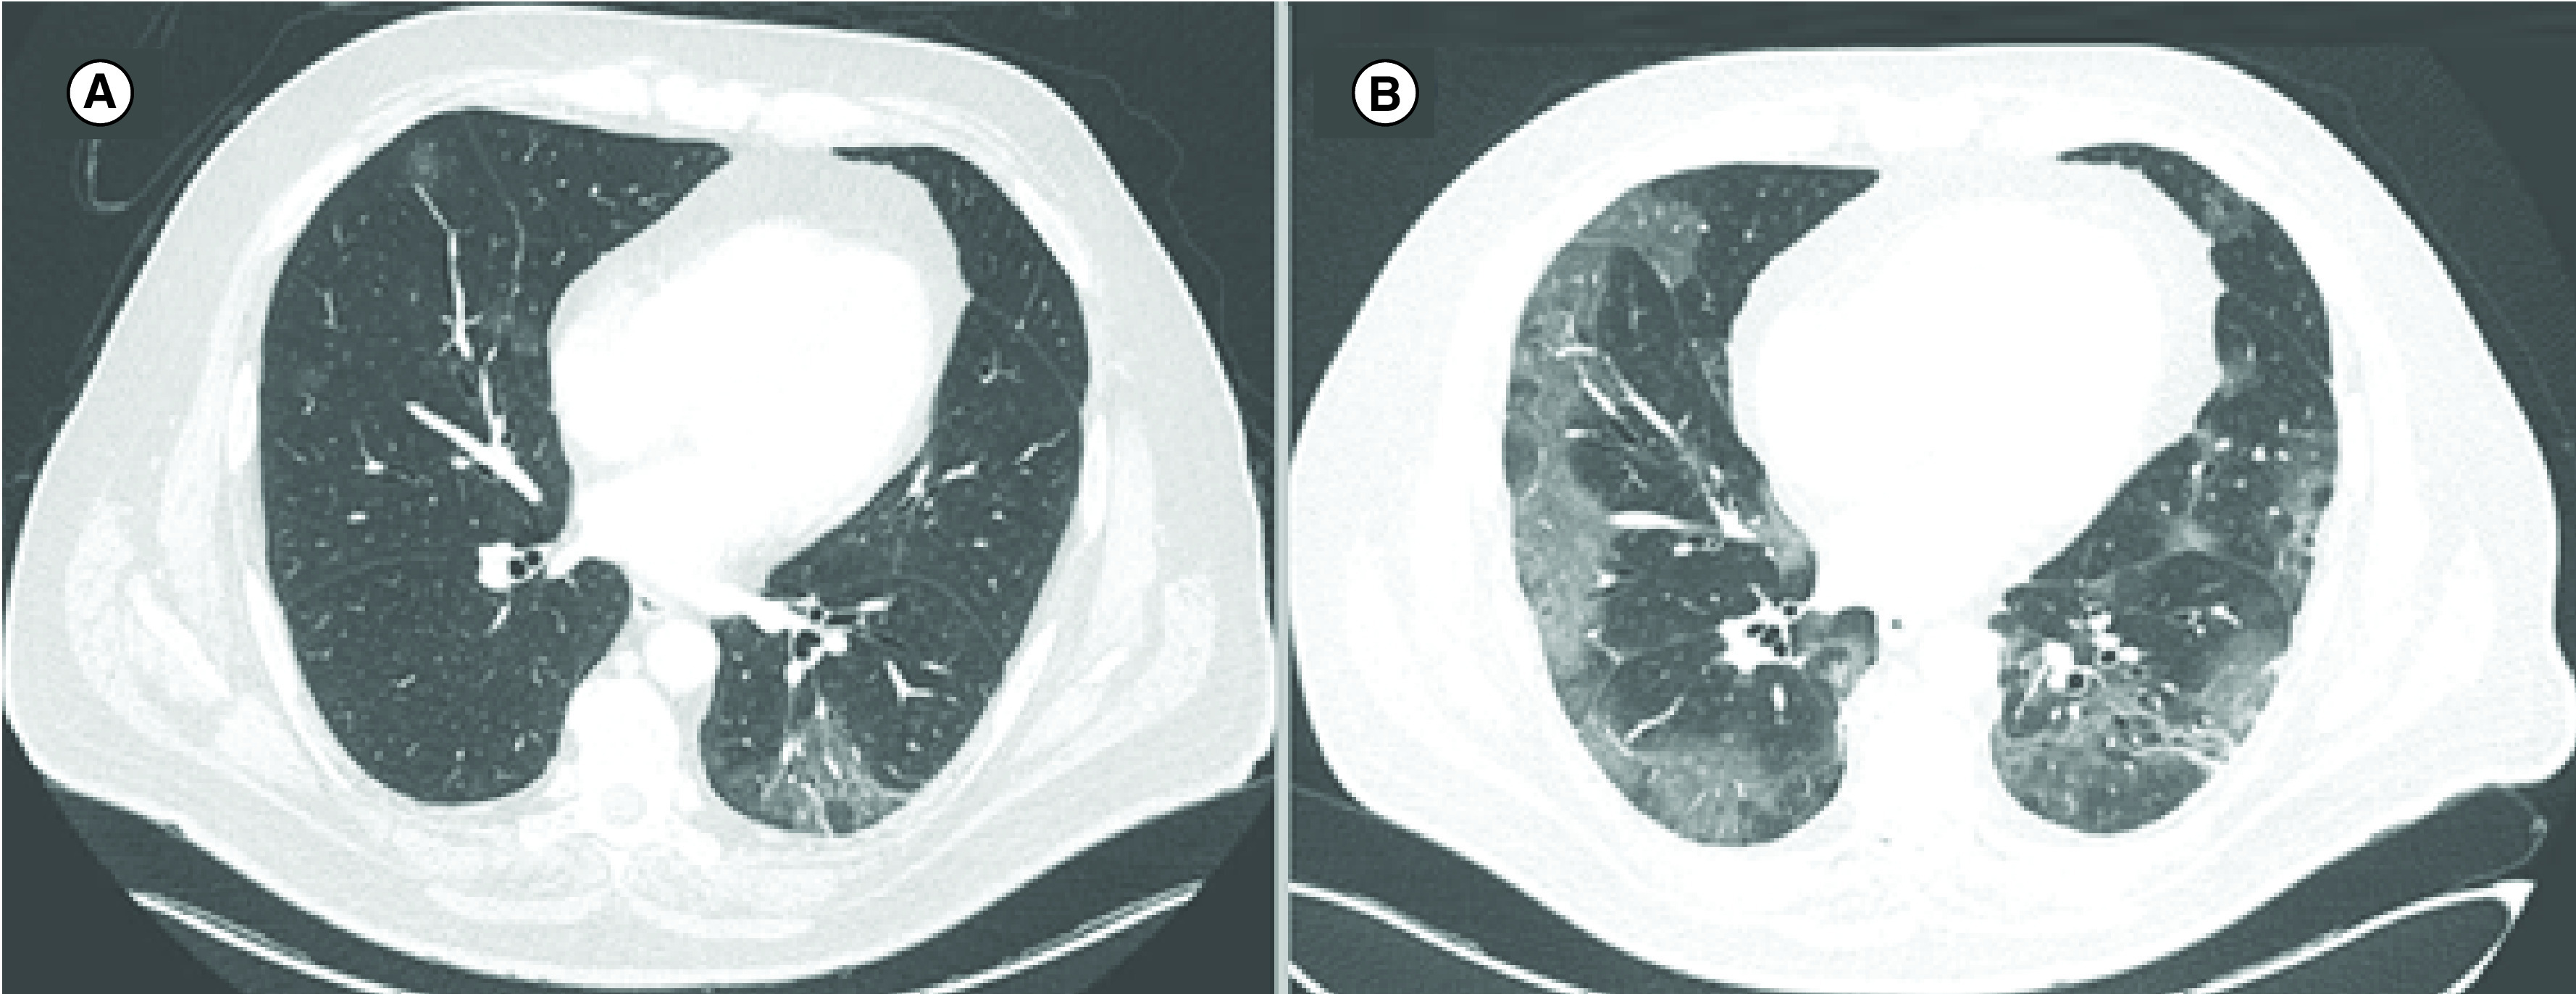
Chest CT. Chest CT showing sparse and bilateral ground-glass opacities, the largest in the left lower lobe, with an inflammatory aspect (A). Deterioration of the ground-glass opacity and bilateral patchy shadowing 7 days later (B).
